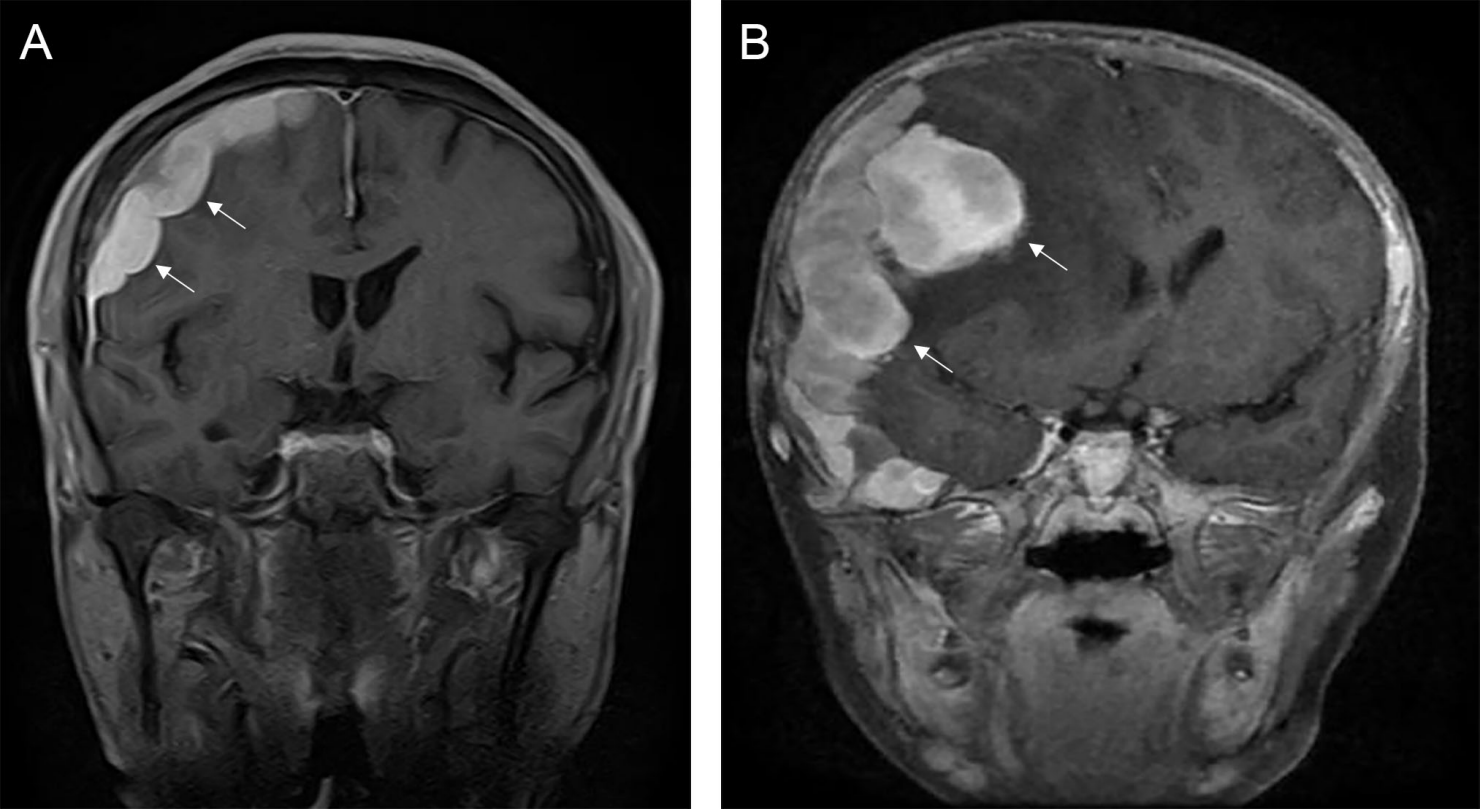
The lobulation sign of CNS-RDD. (A, B) MRI images showed the margin of the lesion is lobulated (arrow) on coronal contrast-enhanced images.
